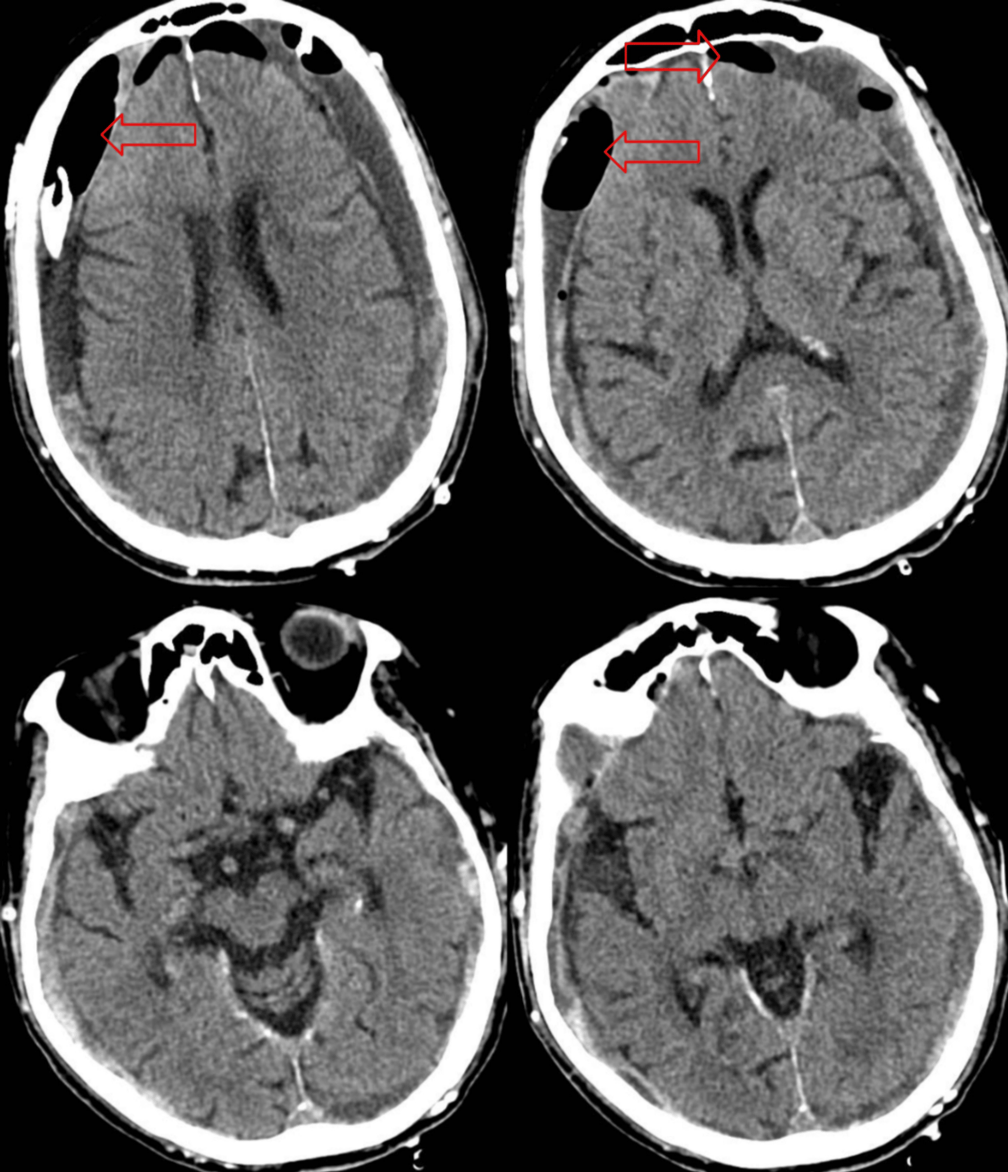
Several slides from the postoperative CT head image study depicting interval bilateral small parietal craniotomies with the placement of bilateral subdural drains since the prior examination with partial evacuation of the significant acute on chronic subdural hematomas, now containing pneumocephalus (red arrows). There is no midline shift. No acute parenchymal hemorrhage. Stable appearance of the brain parenchyma and stable size and configuration of the ventricles and sulci. The basal cisterns are patent.
